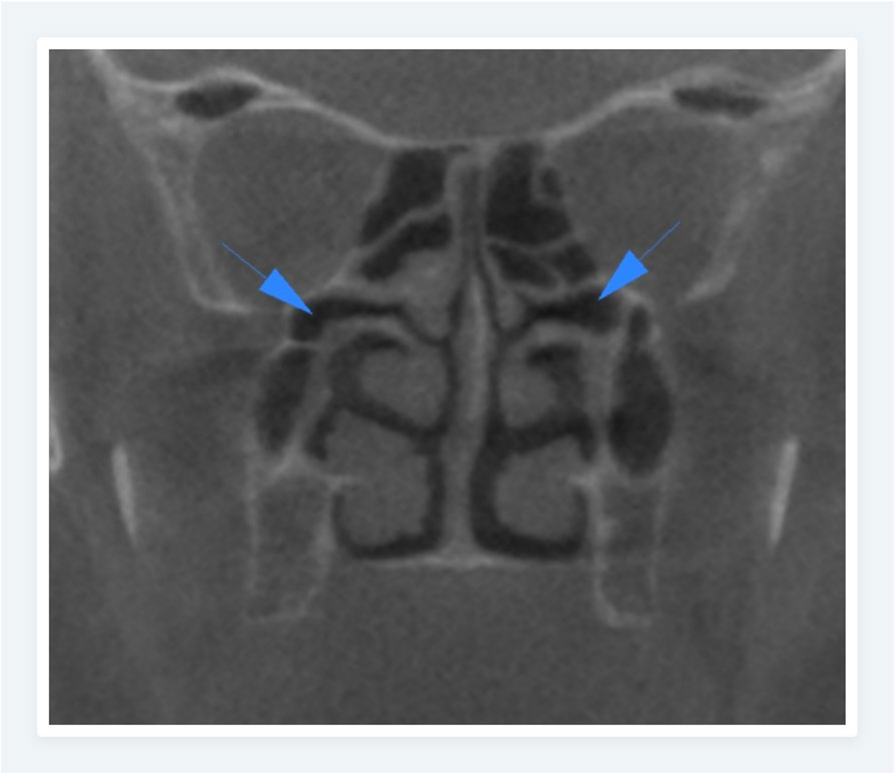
Coronal CBCT image showing the bilateral ethmomaxillary sinuses which drain into the superior meatus
